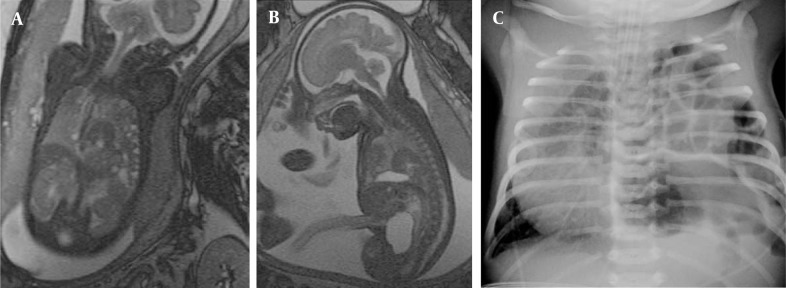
Congenital diaphragmatic hernia in the 35th GW fetus including intestines and spleen on, coronal (A) and sagittal images (B), and postnatal chest x-ray (C).
